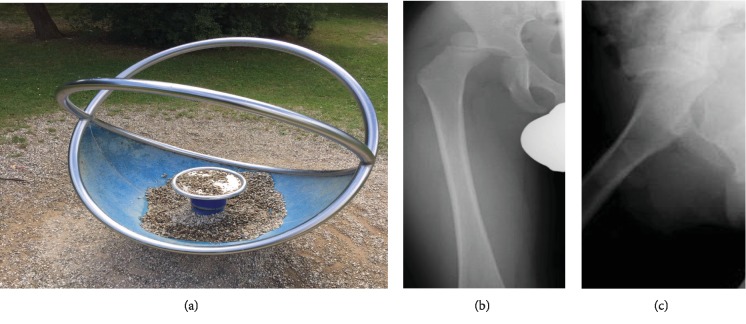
(a–c) Carousel (a); anteroposterior (b) and lateral (c) thigh X-rays showing the posterior luxated hip joint.
